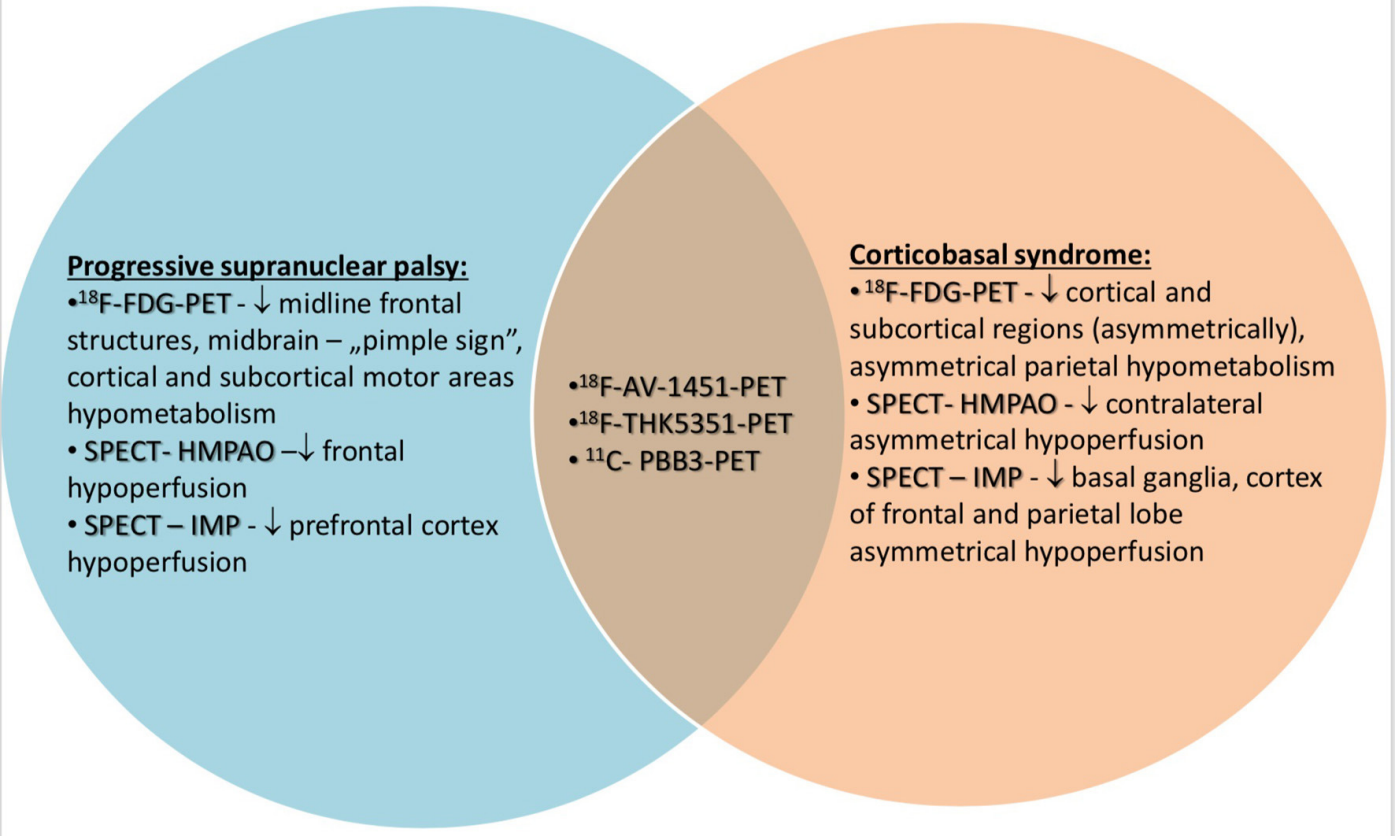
Possibilites and limitations of PET and SPECT in the examination of PSP and CBS.
